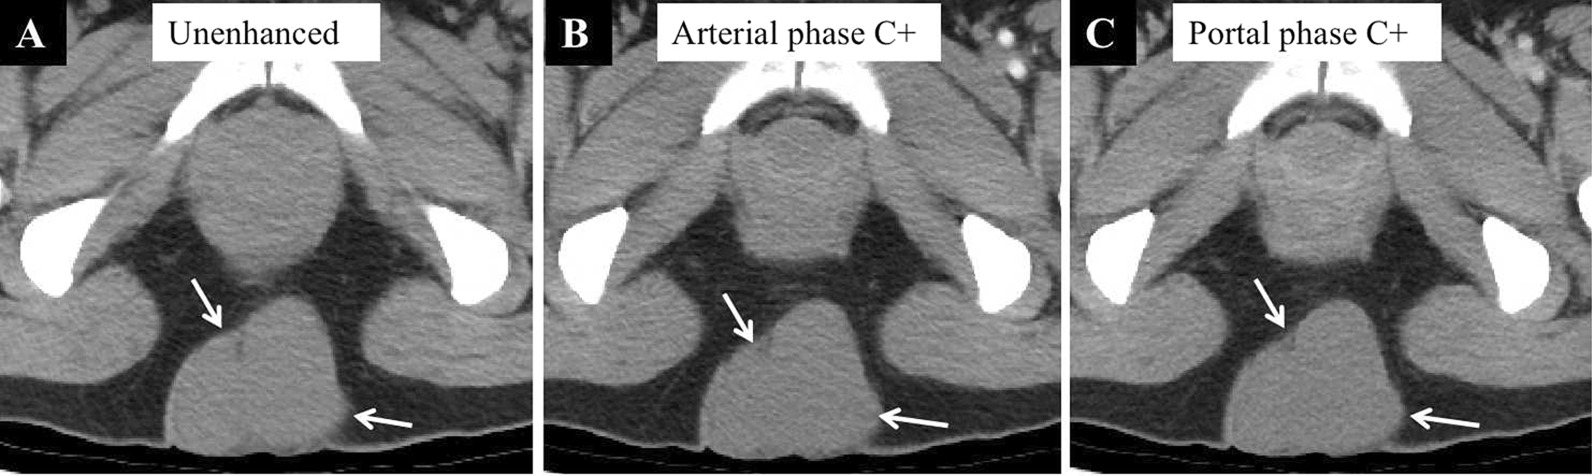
Preoperative computed tomography with and without contrast enhancement. Axial basal unenhanced image (A), arterial phase enhanced image (B) and portal phase enhanced image (C) show a lageniform, homogeneous, soft tissue-attenuation lesion (arrow) in the subcutaneous fatty tissue without calcification or post-contrast enhancement
